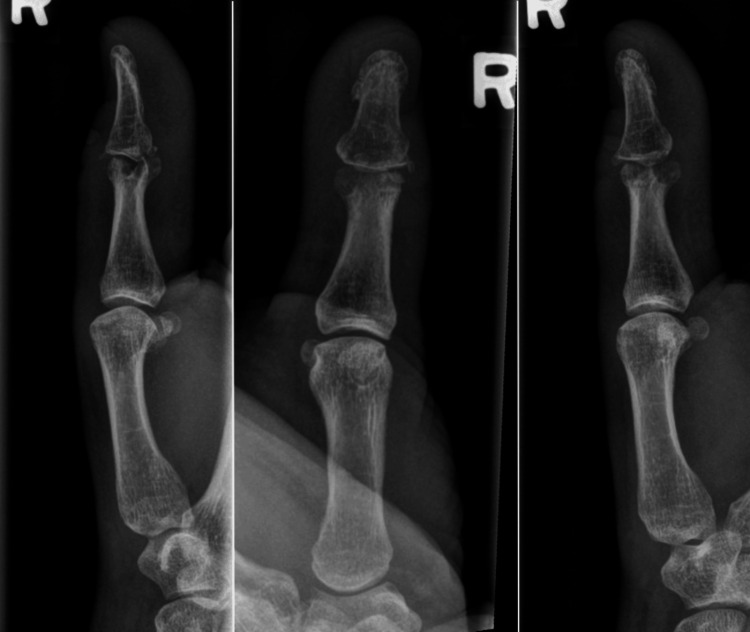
Lateral, anteroposterior, and oblique radiographs of the thumb demonstrating a gouge-like defect in the head of the proximal phalanx with a bony block to the distal phalanx base, preventing active flexion.
